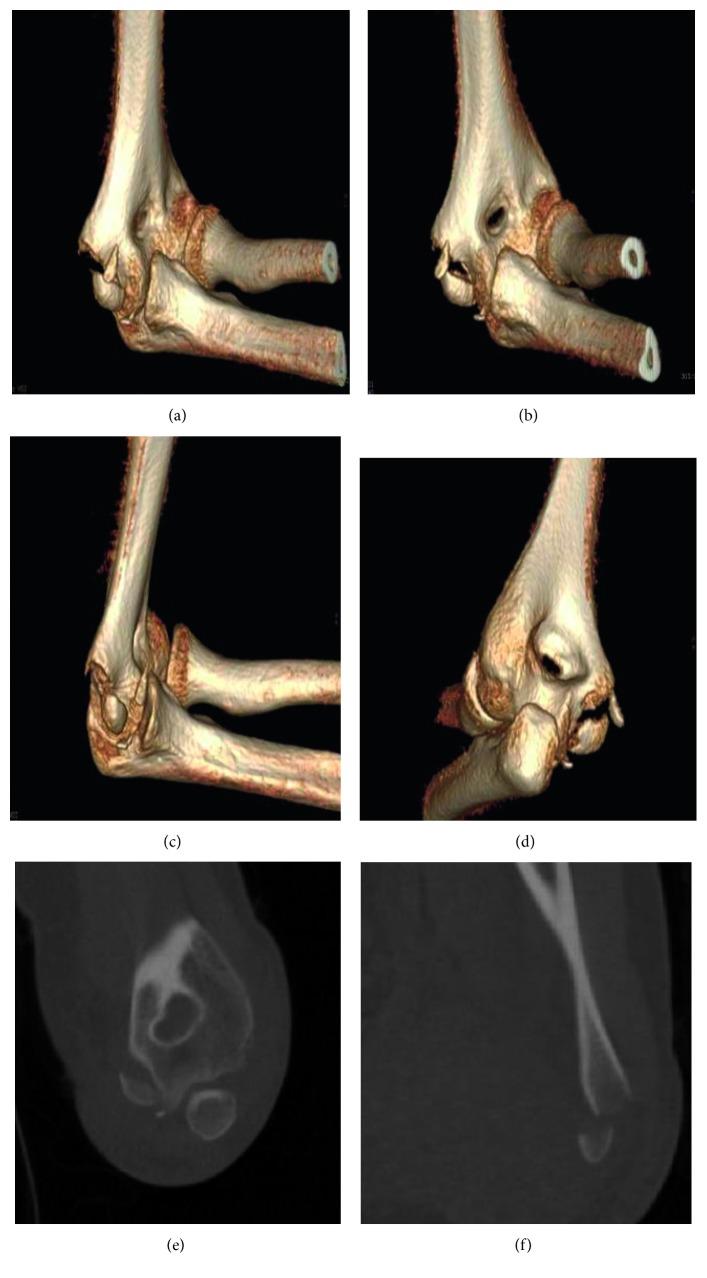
CT scan with 3D reconstruction after closed reduction showing a displaced medial epicondyle avulsion fracture with no other associated bony injury.
